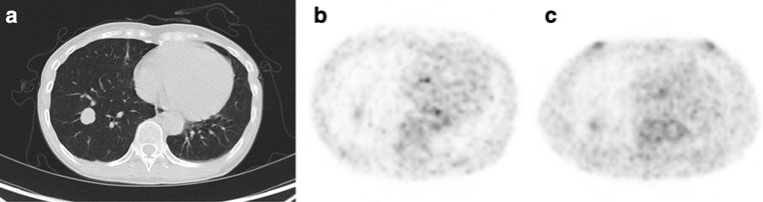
A 60-year-old female patient with an isolated pulmonary lesion in the right lower lobe. DIBH CT (a), DIBH PET (b), and RG PET (c)
